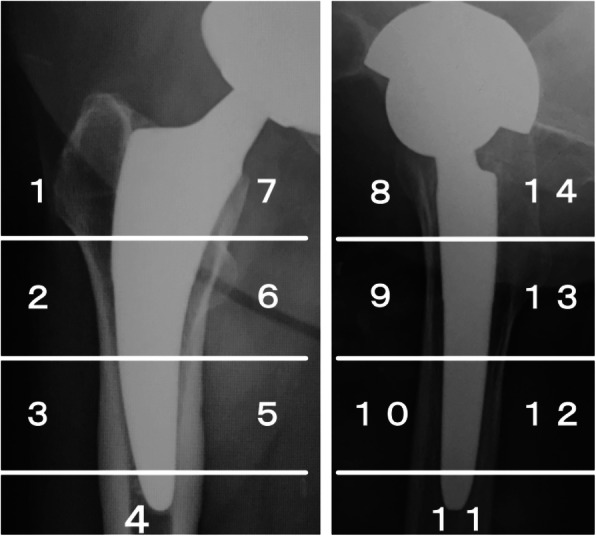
Gruen zones in anteroposterior and lateral radiograph. According to this zoning, radiolucent lines and cortical hypertrophy were evaluated
